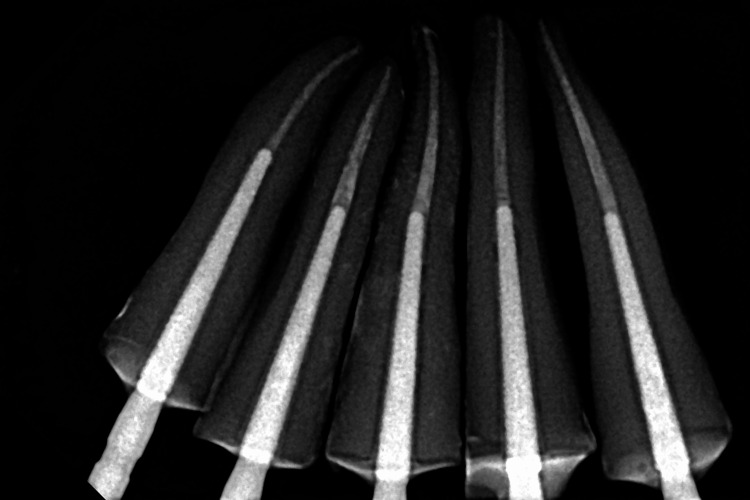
Periapical radiograph after cementation of diamond posts
